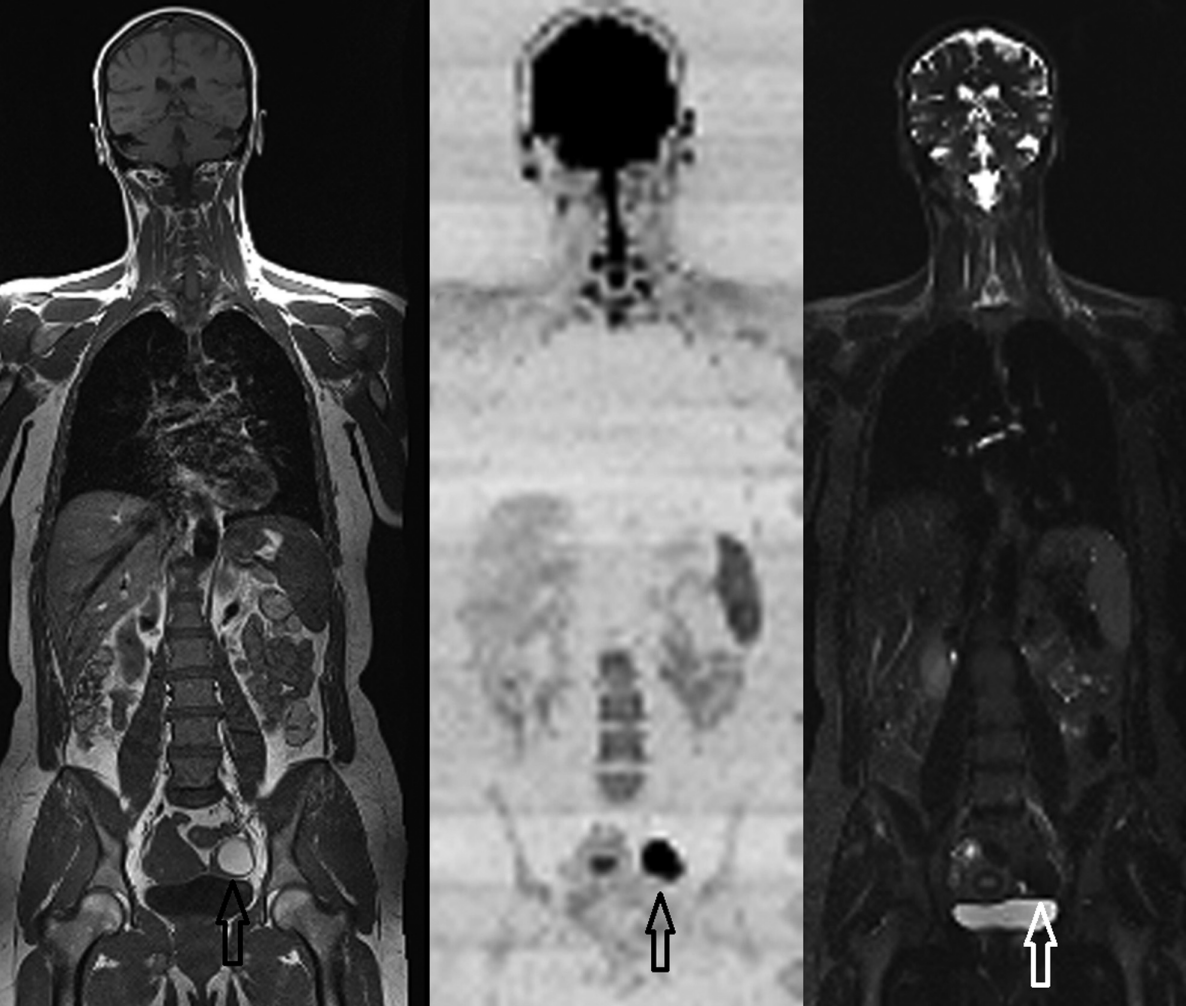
Whole-body coronal T1, DWI (b-500 shown as black on white), and STIR images demonstrating the conspicuity of the left adnexal subacute hemorrhage in an endometrioma due to the paramagnetic effects and restricted diffusion of blood degradation products.
